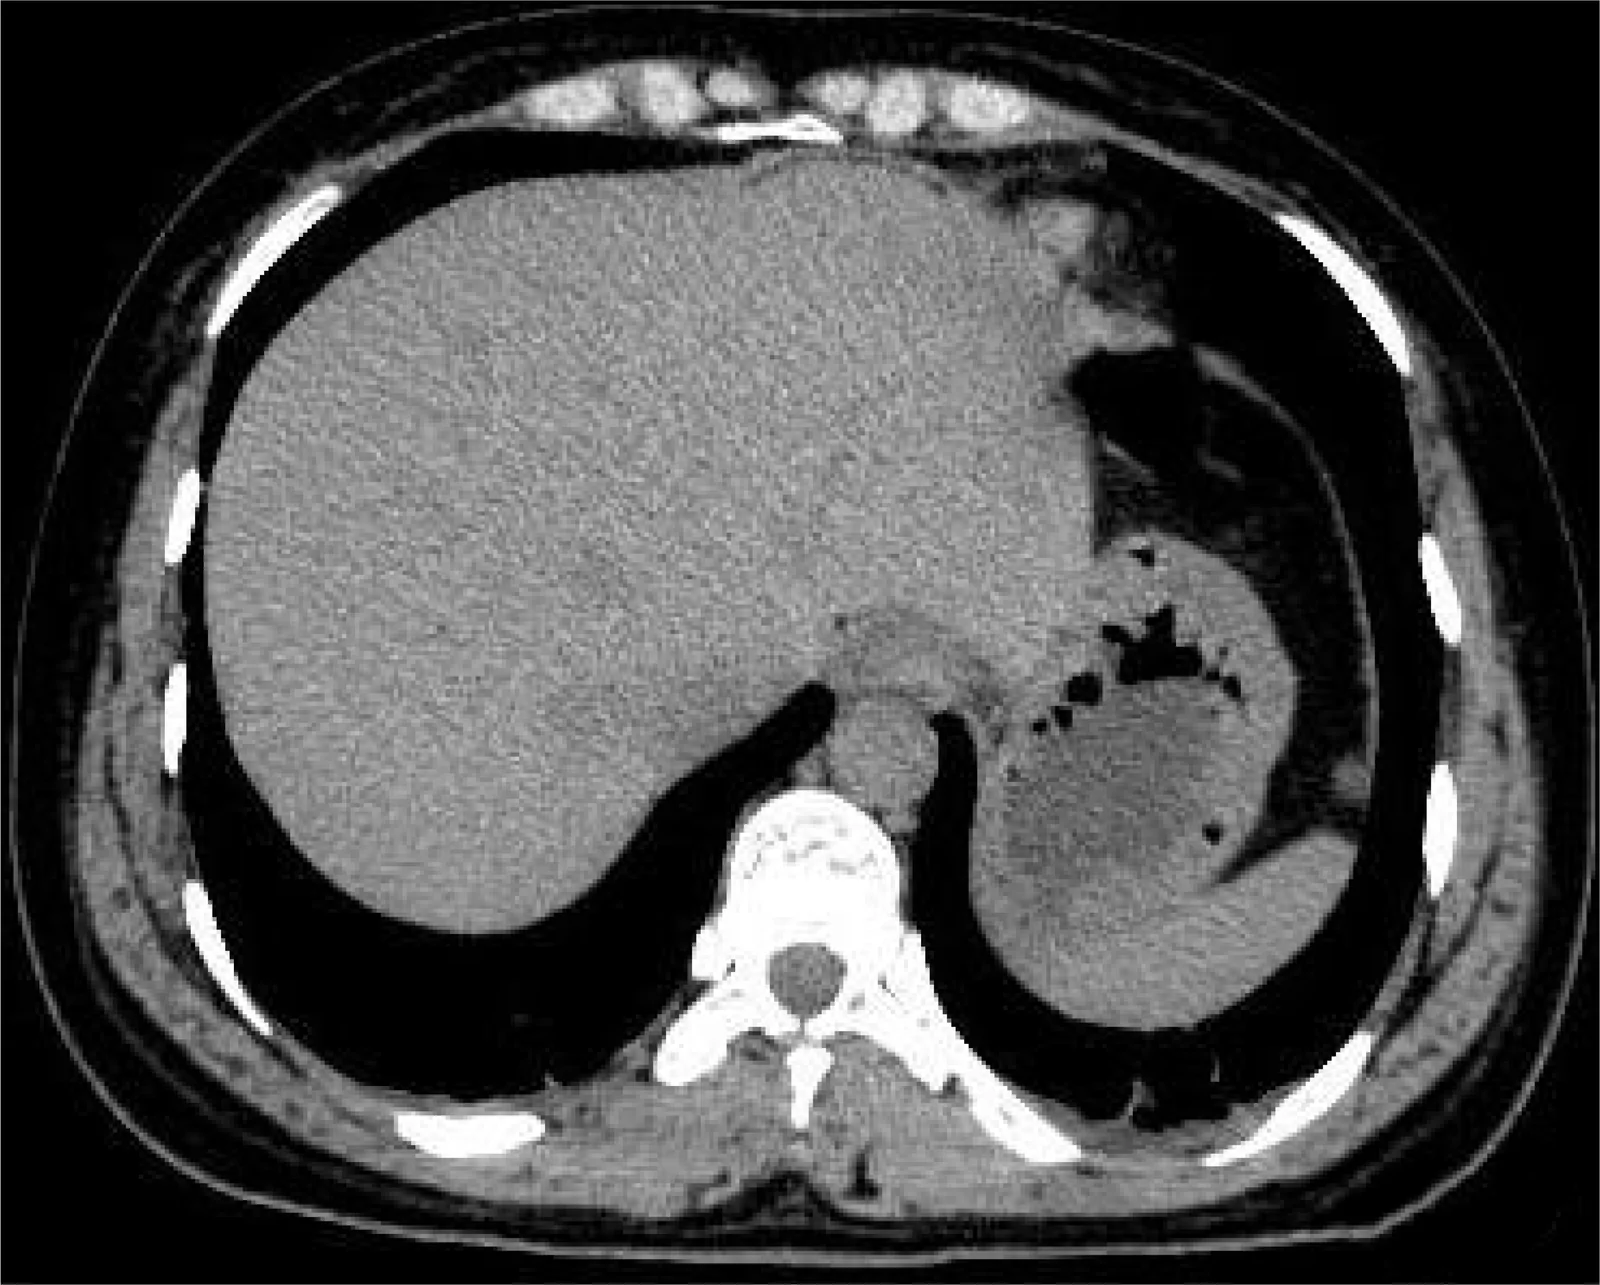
Follow-up abdominal CT shows that the previously observed heterogeneous, irregular mass-like density shadow in the stomach has now disappeared.
